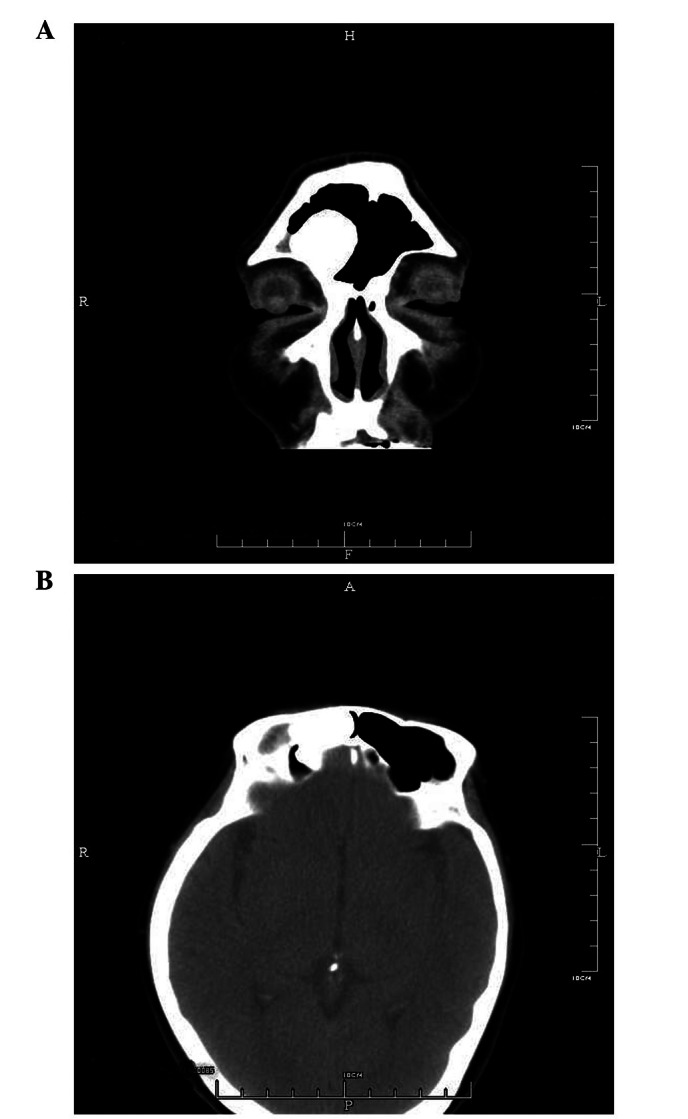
(A) Computed tomography (CT) scans of the sinuses were performed and revealed a 3.5×3-cm extremely dense bony mass with inflammations in the right frontal sinus, approaching the edge of the sinus. (B) An osseous lesion in the right frontal sinus in axial CT images.
